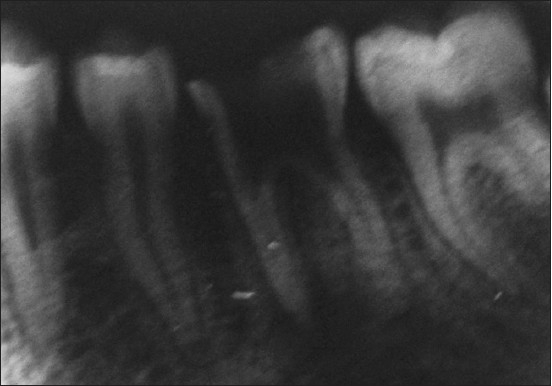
Radiographic evidence of perforation defect extending into the furcation area
